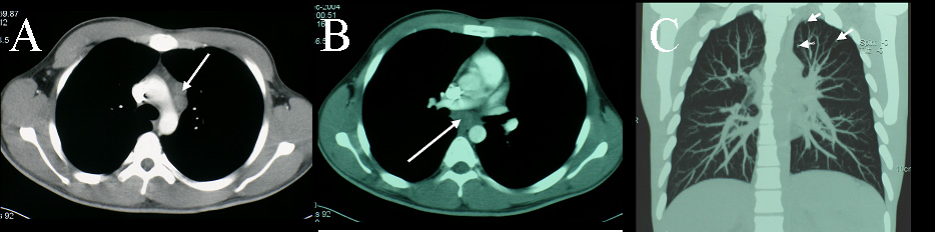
Multiplanar chest CT. This demonstrates mediastinal lymphadenopathy and subtle pulmonary involvement. A. Contrast enhanced axial slice, showing enlarged lymph nodes (arrowed) in the para-aortic window. B. Axial slice showing subcarinal lymphadenopathy (arrowed). C. Coronal slice, showing small nodules in the left upper lobe (arrowed).
